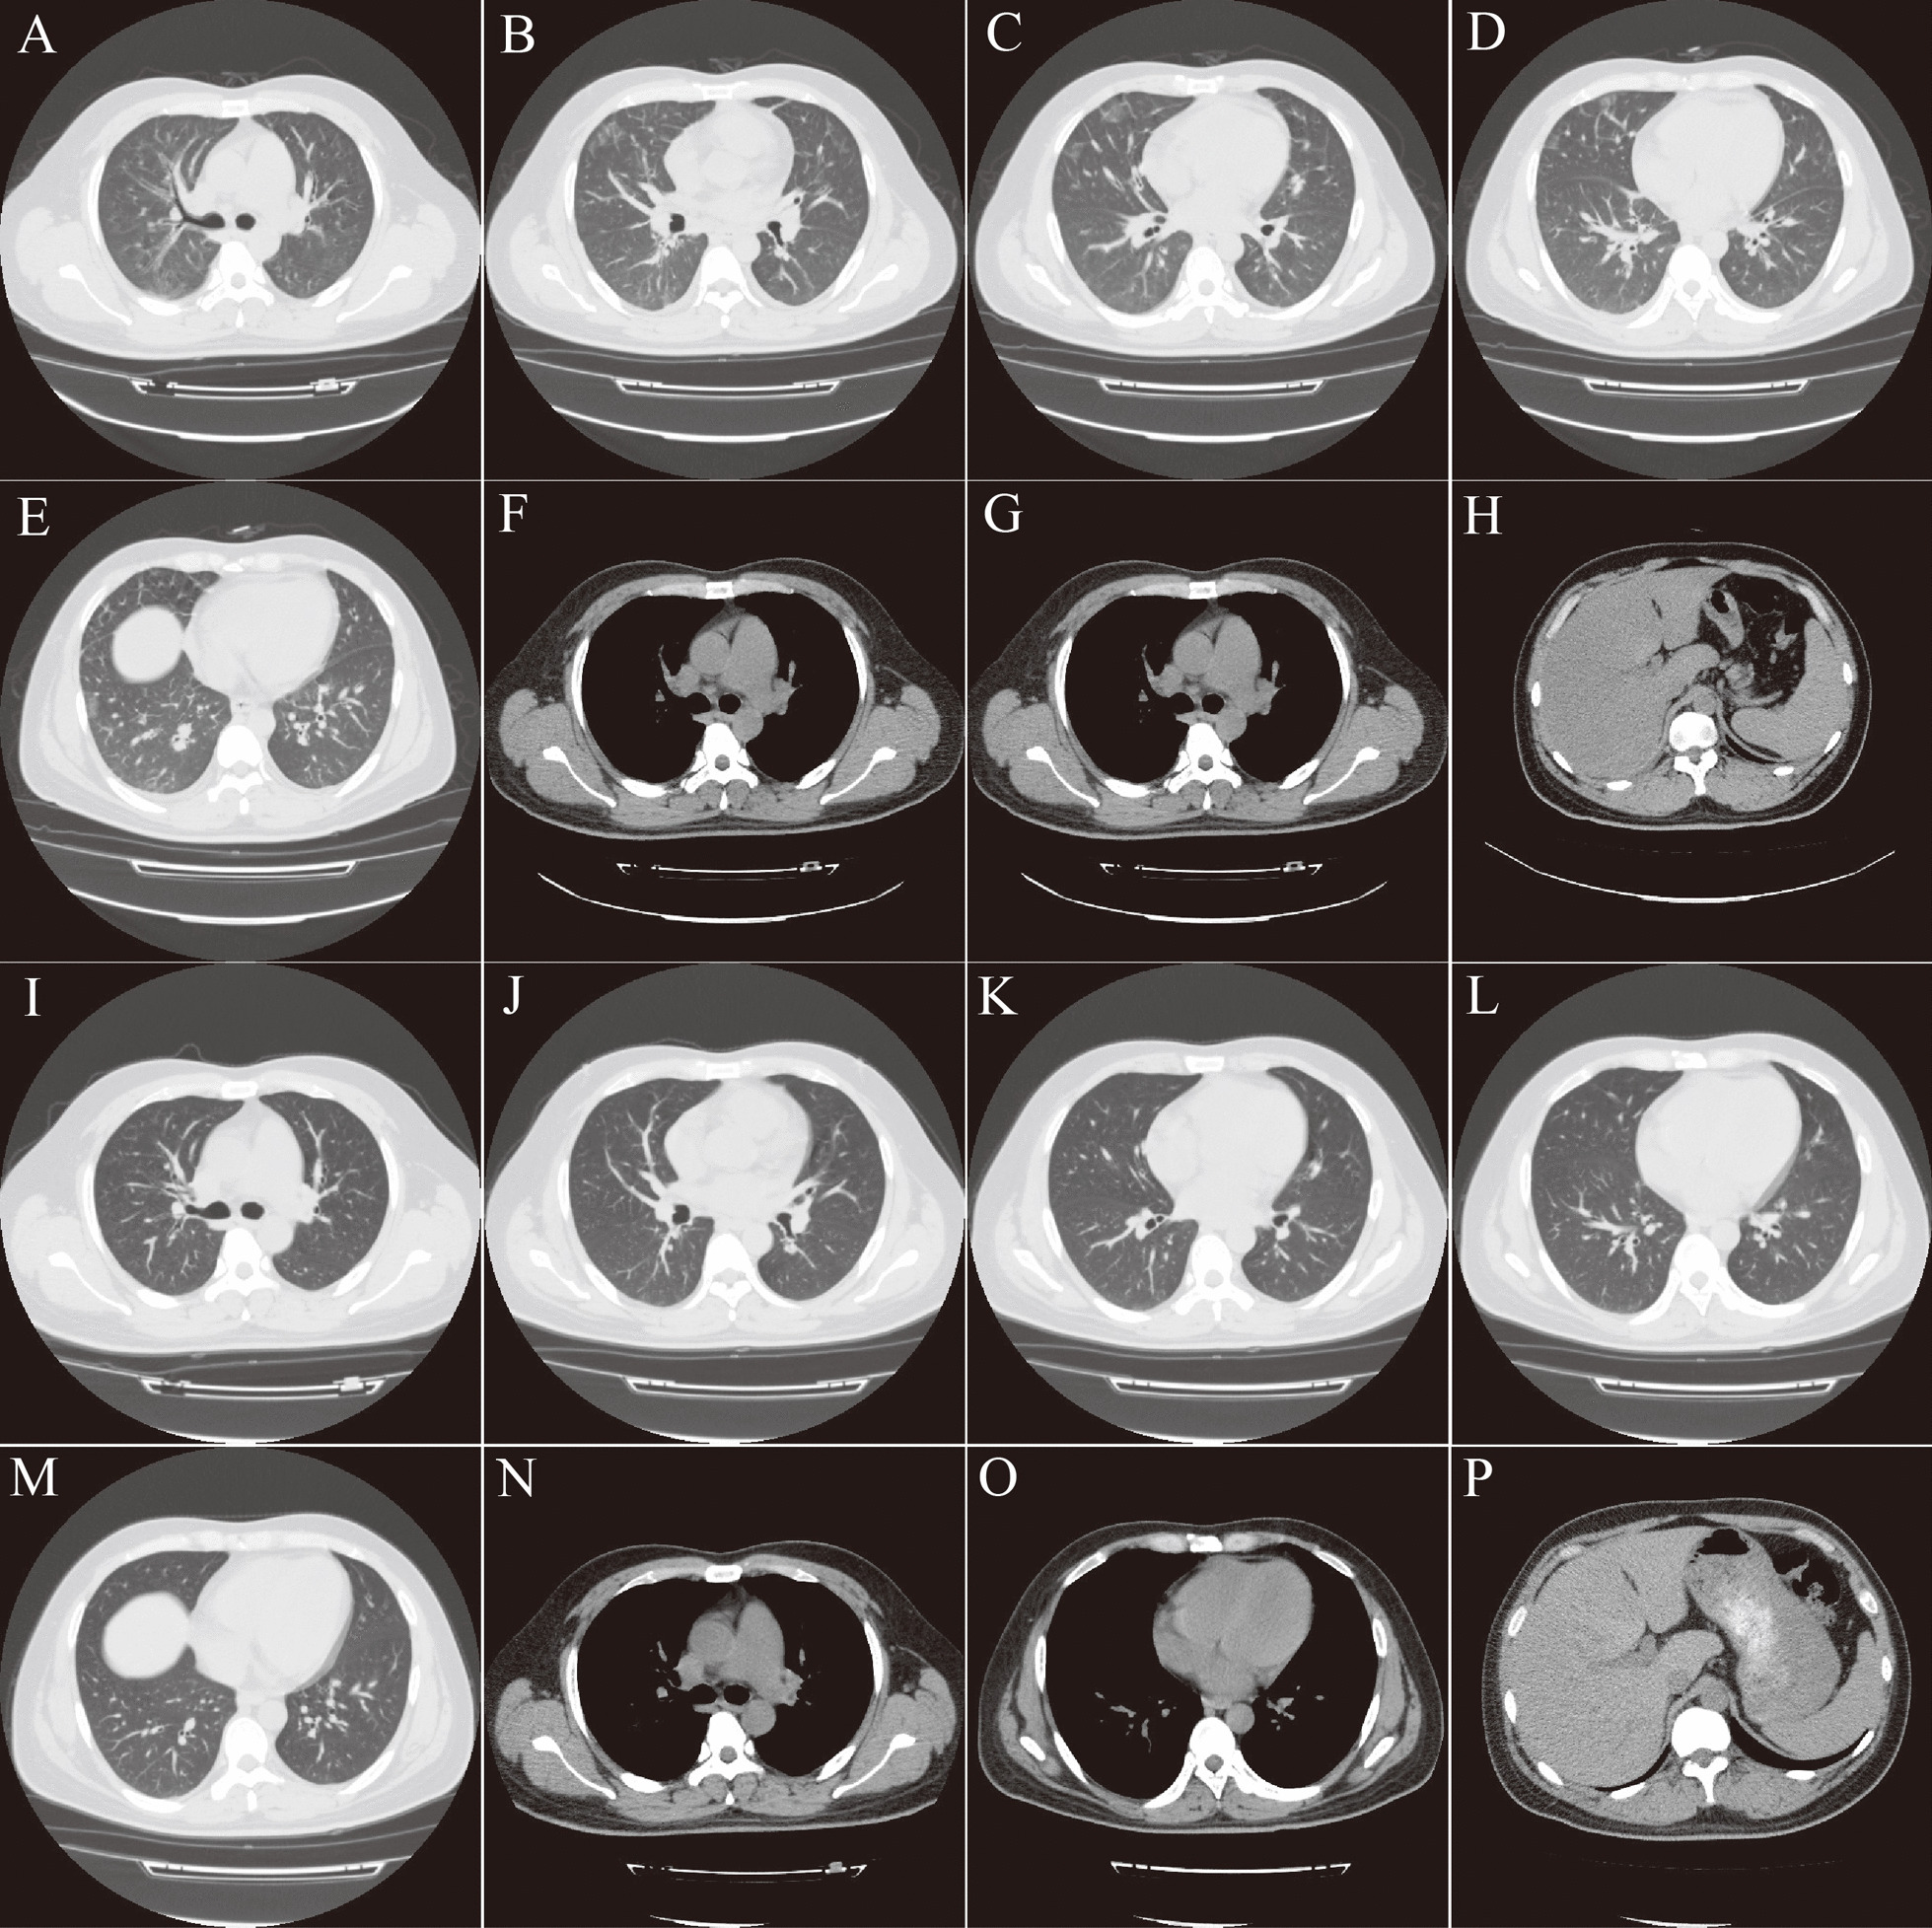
Presentation of Chest and Abdomen computed tomography (CT) findings. Chest CT showed thickened bronchial walls with surrounding interstitium in both lungs, double-track signs (A), thickened interlobular septa, multiple ground-glass opacities in the periphery of both lungs (B–E), widened pulmonary artery segment (F), a small amount of pleural effusion on both sides and pericardial effusion (G). Abdomen CT showed severe fatty liver and splenomegaly (H). After 2-week chest CT indicated significant improvement of pulmonary lesions (I–M), normal pulmonary artery segment is visible (N), pleural effusion and pericardial effusion disappeared (O), fatty liver and normal spleen (P)
